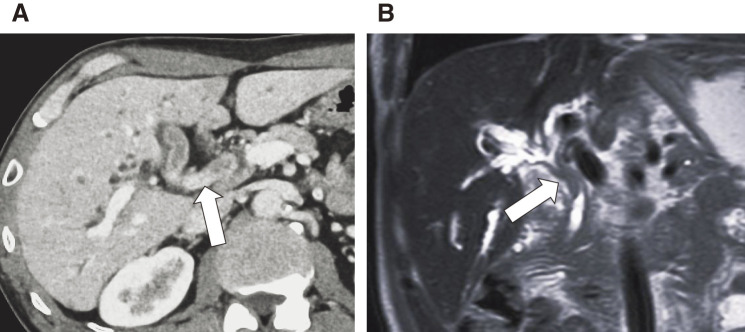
Initial computed tomography (A) and magnetic resonance imaging (B) scans at the time of diagnosis. The images demonstrate a soft tissue mass in the common bile duct at the level of the confluence of the choledochal ducts (white arrows).
